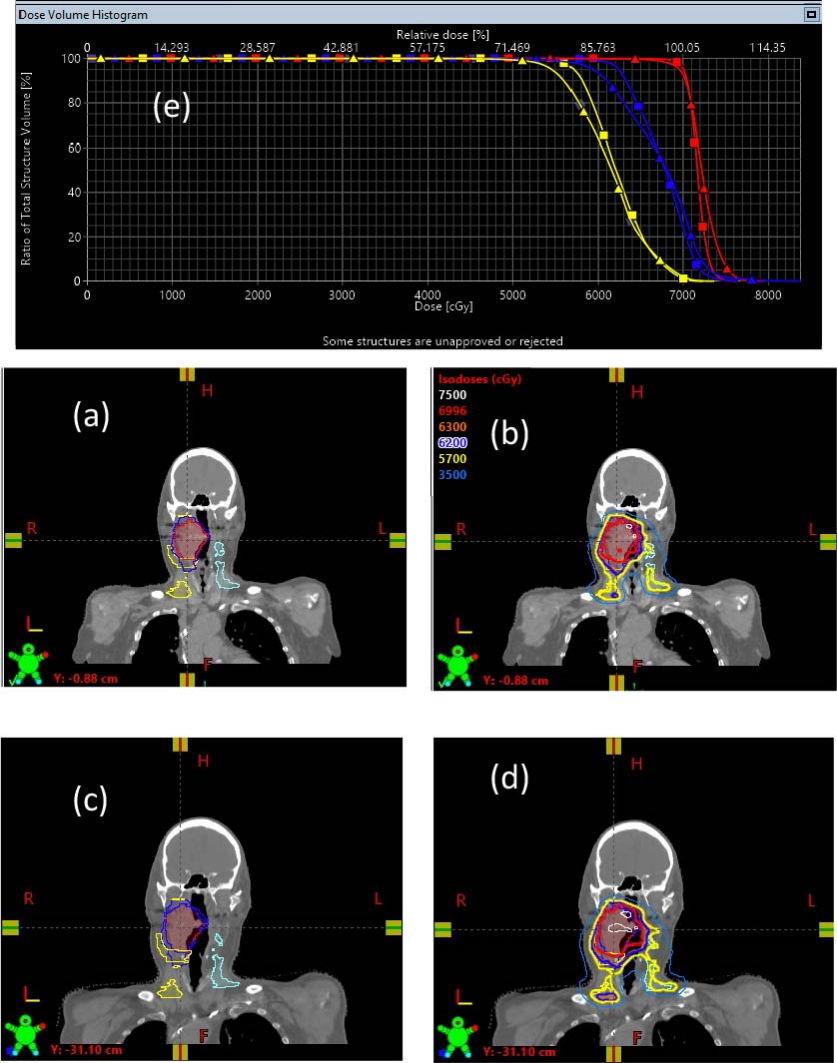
An example of verification planning process that triggered adaptive planning. (a and c) CT images and target contours (red: CTV 6996; blue: CTV 6300; yellow CTV 5700 [right]; cyan: CTV 5700 [left]) at planning (a) and verification (c). Target shrinkage can be seen by comparing (a) and (c). (b and d) Dose distributions using CT (a) and (c). (e) Dose-volume histograms at planning (square) and verification (triangle). Abbreviations: CT, computed tomography; CTV, clinical target volume.
